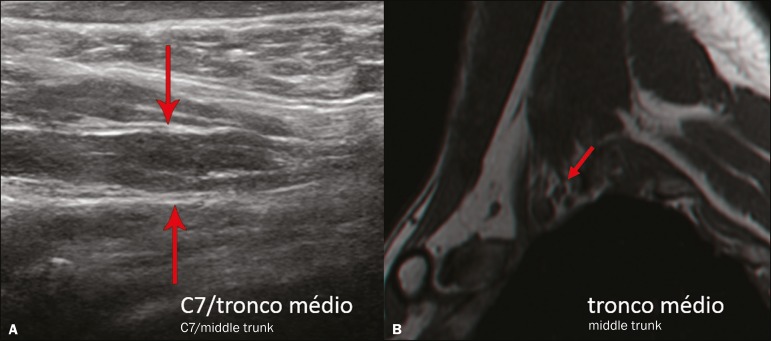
A: Ultrasound in the longitudinal plane showing the C7
